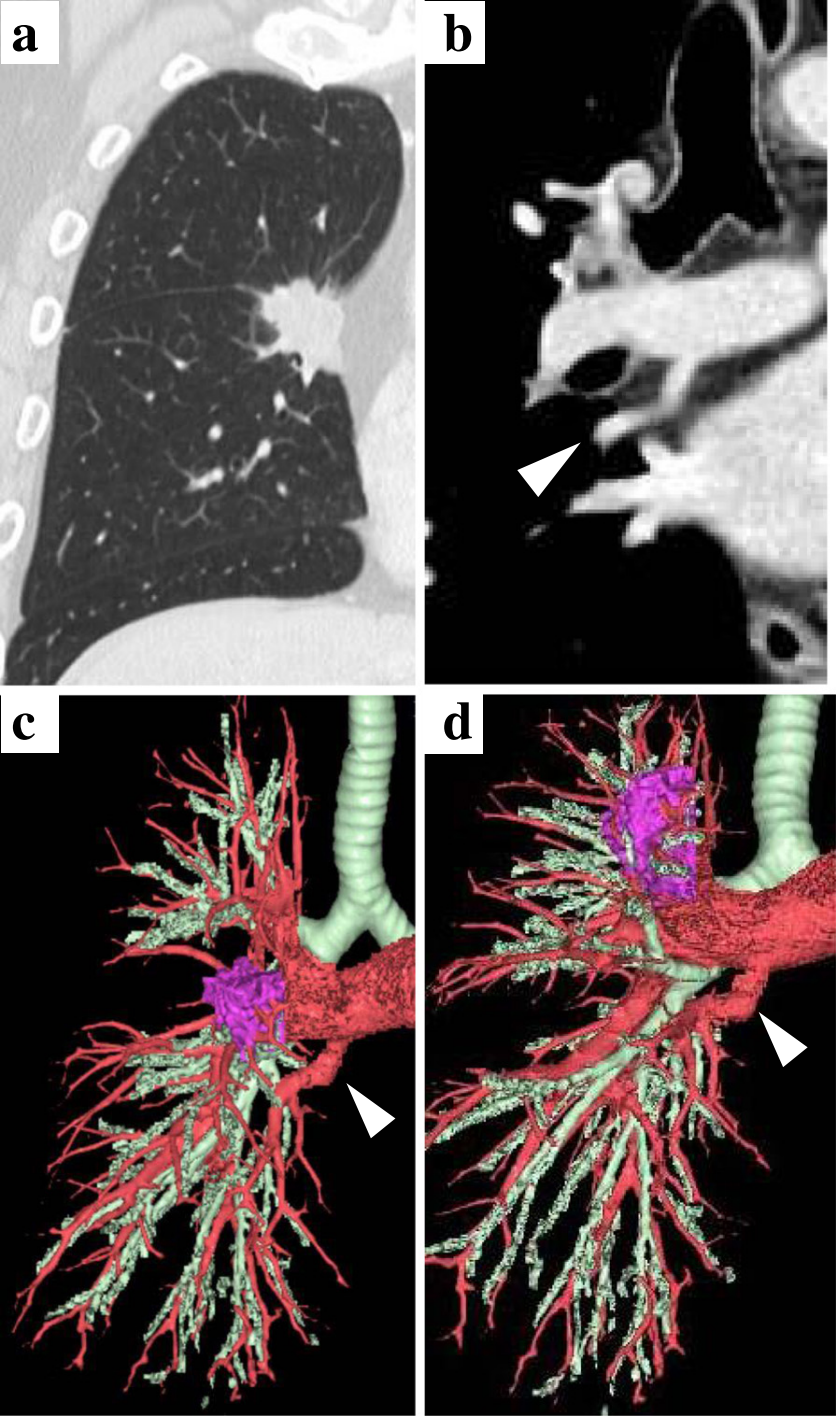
The tumor exists in the right middle lobe and is suspected the infiltration into the mediastinal fat (T4) in the preoperative chest CT (a). And, the coronal CT shows abnormal A7 (◁) branching from right main pulmonary artery (b). The three-dimensional computed tomography is the similar findings (c). If we look from the bottom a little, branching from main PA was easier to understand (d)
